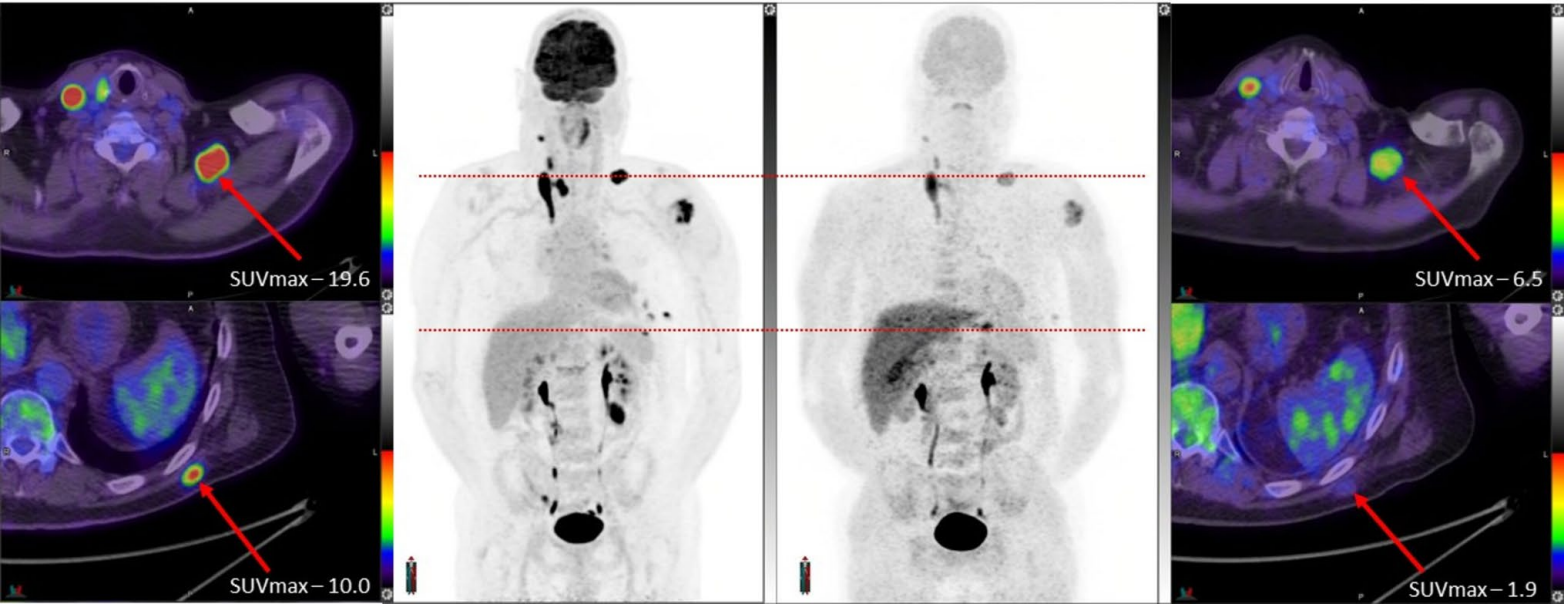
(Patient #9): Fused PET/CT through the lower neck (above) and lower thorax (below) are indicated on the respective MIP images for [18 F]FDG on the left and [18 F]MEL050 on the right. Although [18 F]MEL050 uptake is observed in multiple sites, the uptake in this case is consistently lower than for [18 F]FDG and a subcutaneous lesion in the left lower back was rated as negative on blinded reading
